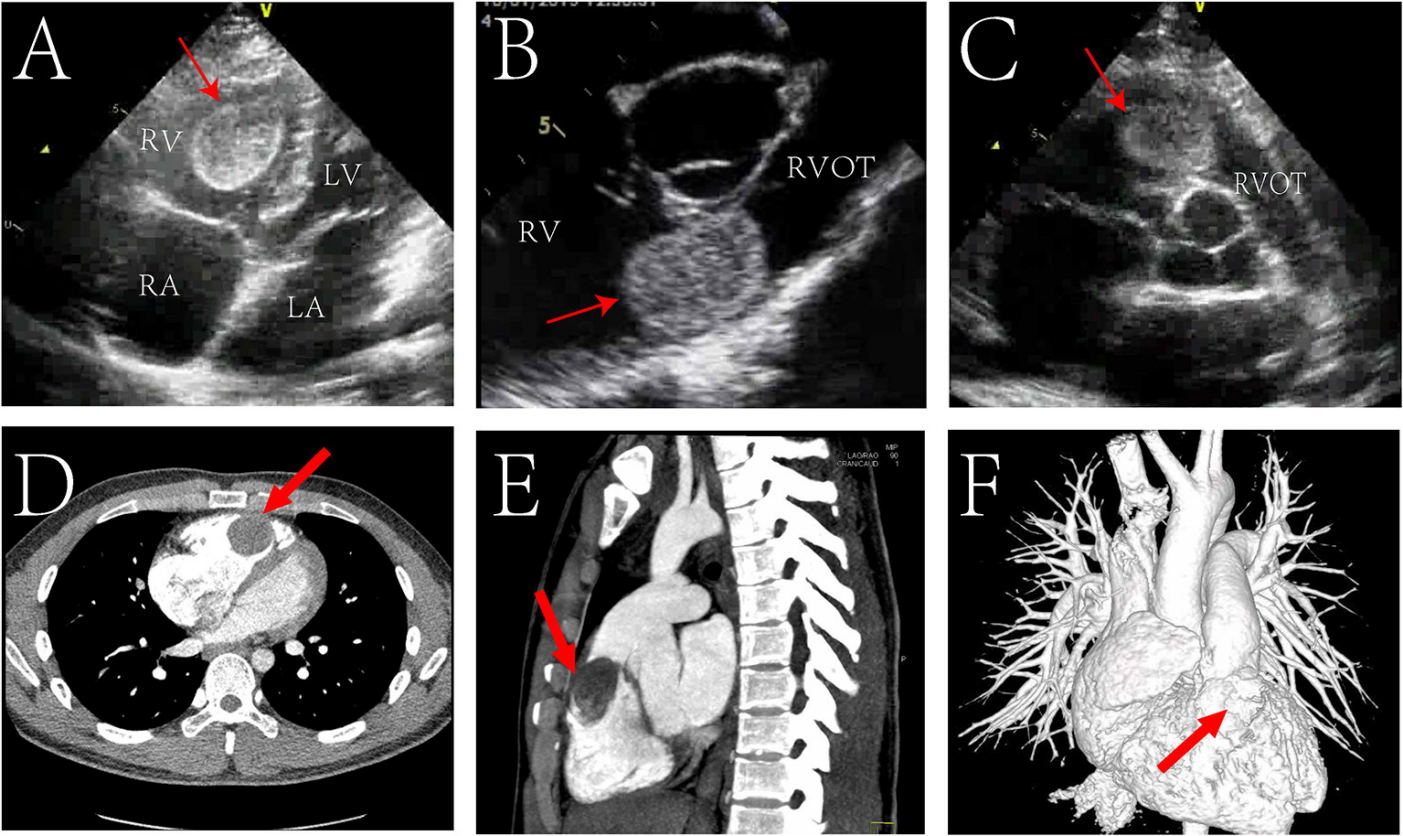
Echocardiogram pre-operatively showing a mass (red arrow) located in the in the right ventricular outflow tract (RVOT) in transthoracic four chamber cross section (A), transthoracic short axis section of aorta (B), transesophageal short axis section of aorta (C). Preoperative cardiac Computed Tomography (CT) showed a mass (red arrow) in the RVOT in transverse view (D), sagittal view (E); CT angiography (CTA) showed a filling defect (red arrow) in the right ventricular outflow tract (F). RV, right ventricle; LV, left ventricle; RA, right atrium; LA, left atrium.
